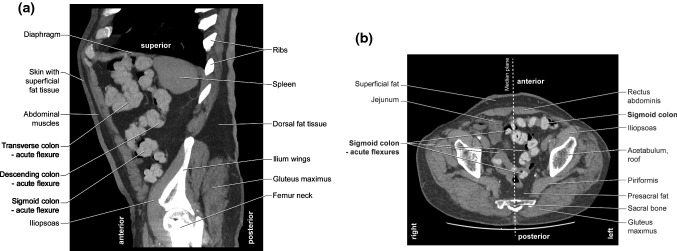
CT scans (tracing—main features). Number of acute colon flexions—20. Patient RW. M, age 83 years, weight 92 kg, height 178 cm, BMI 29. Date of examination: 30.11.2018. A Sagittal plane. There are 3 acute colonic flexures on the scan (1 in the sigmoid colon, 1 in the descending colon, and 1 in the transverse colon) B Horizontal plane—focused on the acute flexures in the sigmoid colon (4 flexures are seen on this scan)
